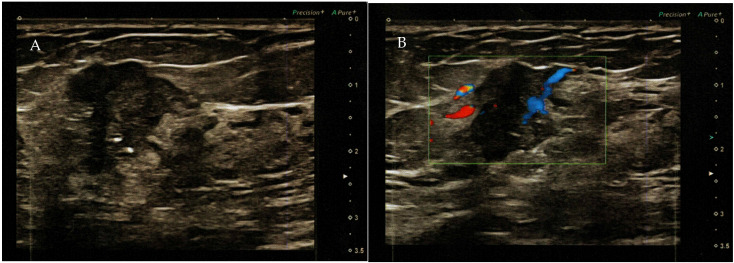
(A) Color ultrasound revealed a nodule in the right upper outer quadrant of the breast with disordered structure, increased echogenicity, uneven distribution of mammary glands, unclear boundary, irregular shape, and a strong echogenic spotted hypoechoic nodule with a diameter of 2.2 × 1.4 cm (BI RADS—4B). (B) Mixed blood flow signals in the tumor.
